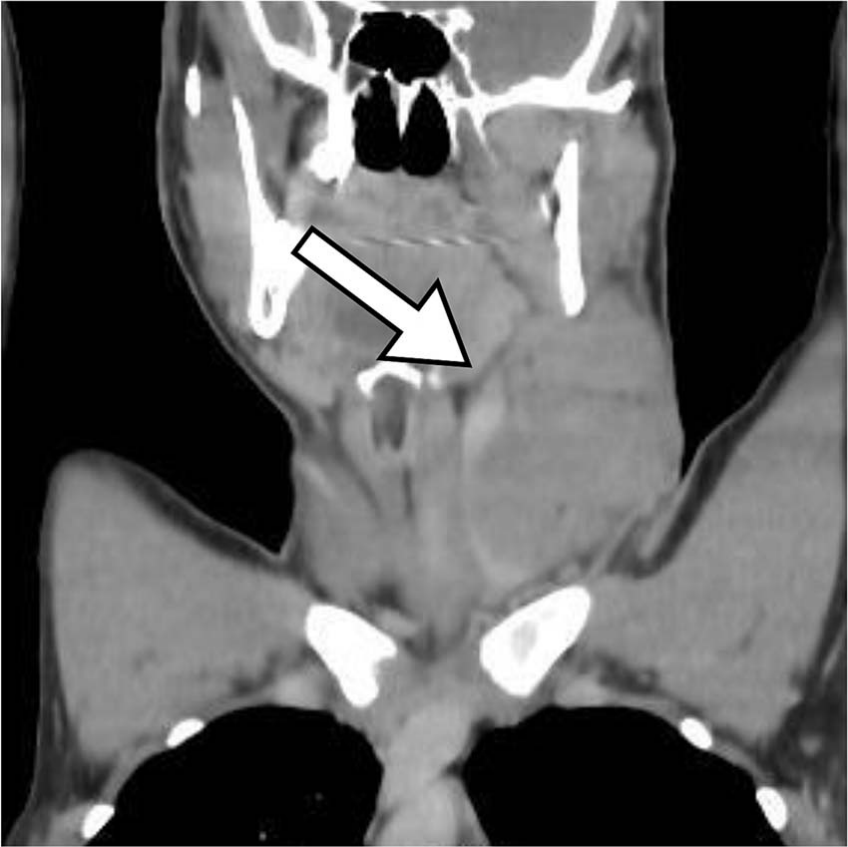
Coronal section of CT -neck showing supraclavicular lymph node (white arrow).
